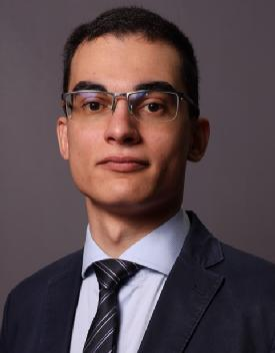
André Vaz is a cardiovascular radiologist based in São Paulo, Brazil. He completed his Fellowship in Cardiovascular CT and MRI at the Instituto do Coração do Hospital de Clínicas da Faculdade de Medicina da Universidade de São Paulo, under the supervision of Carlos Eduardo Rochitte. Prior to this, he earned a Master’s Degree in Internal Medicine from the Universidade Federal do Paraná and completed fellowships in Pediatric Radiology at Hospital Pequeno Príncipe. He obtained his Doctor of Medicine degree from the Universidade Federal de Santa Catarina. He is currently involved in research into congenital heart disease, 3D printing, and genetic cardiomyopathies.
